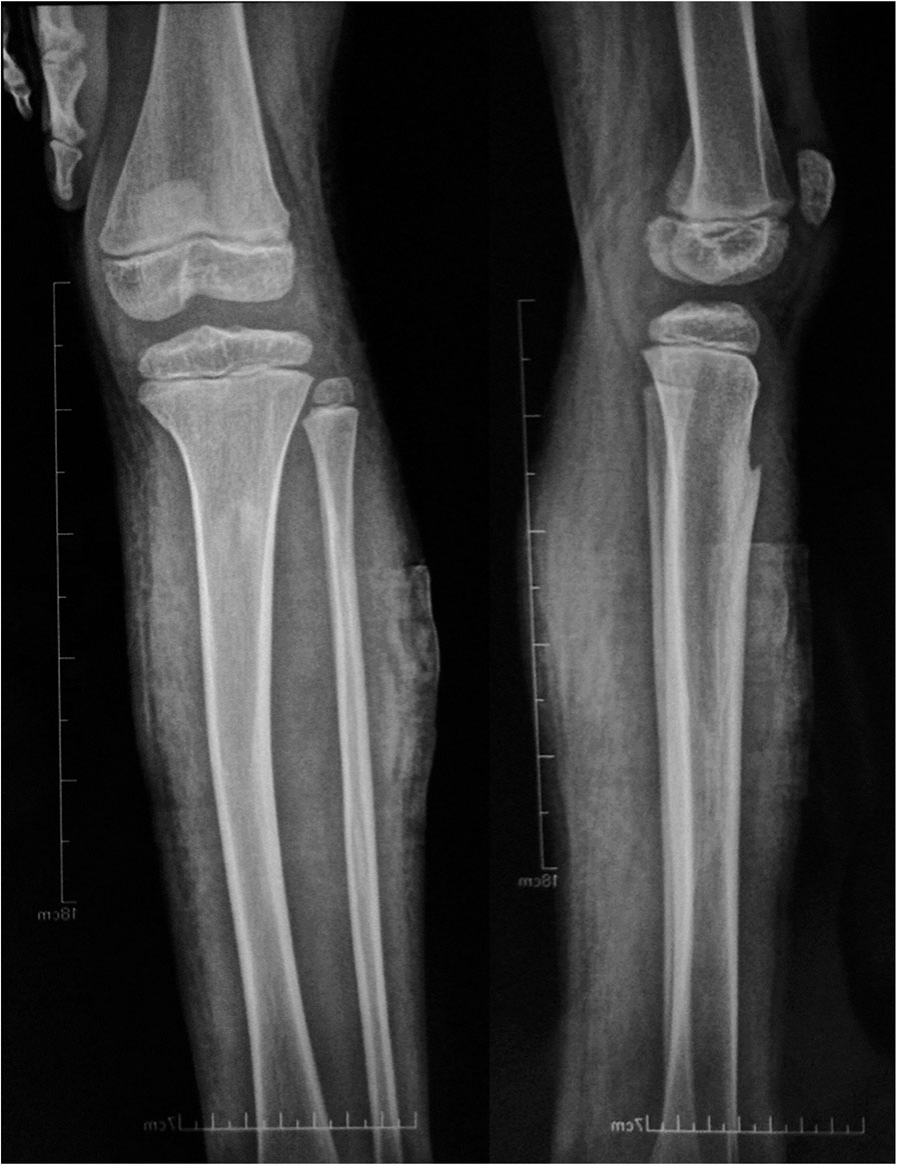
Initial radiograph of the tibiofibular shows no abnormalities
